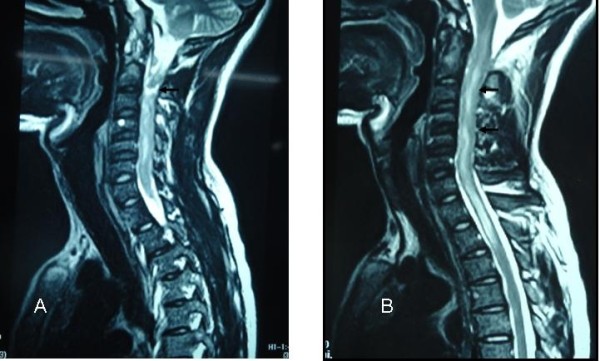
T2-weighted magnetic resonance imaging revealing intra-medullary tuberculoma. Sagittal sections; a hyper-intense lesion is visible along the whole cervical region (A,B) (arrows) and upper thoracic region (B).
